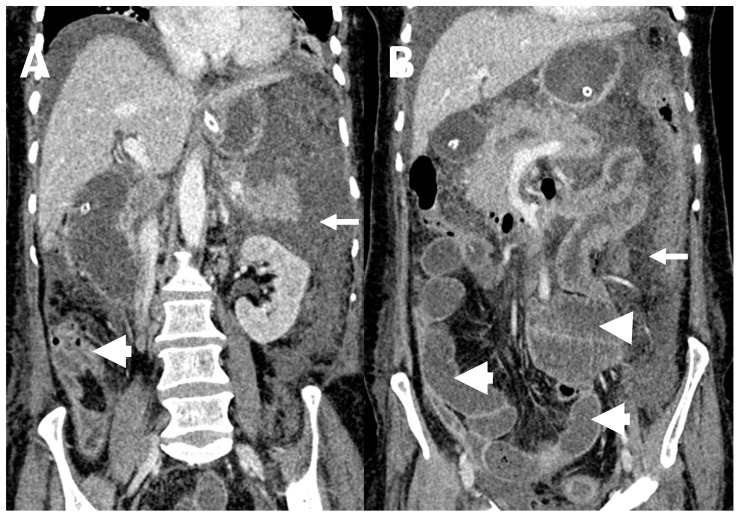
Peritoneal tuberculosis in a 44-year-old male. Coronal contrast enhanced CT images show moderate ascites (arrows, (A)) with diffuse omental stranding (arrow, (B)). Also note, associated small bowel dilatation (thick arrow, (B)) and mural thickening at the terminal ileum and ileocaecal junction (thick arrow, (A)).
